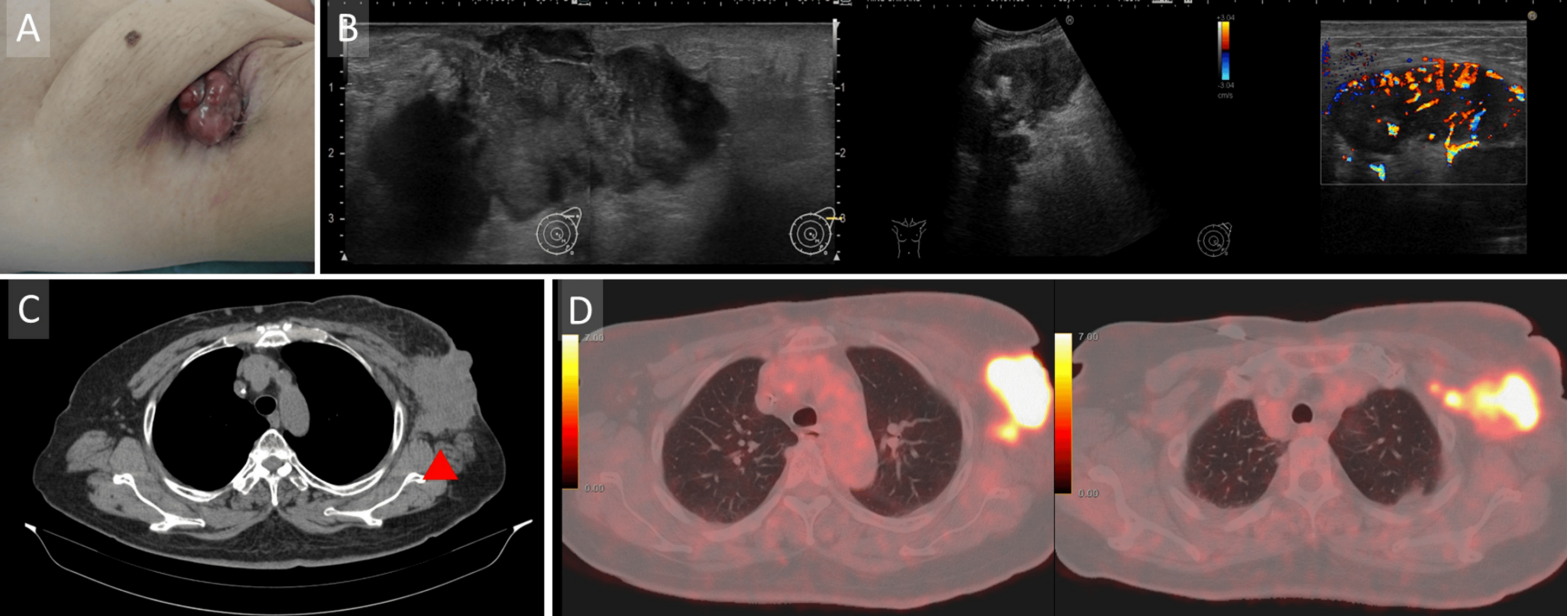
Preoperative examination (A) Clinical picture of the left axillary tumor, showing the tumor protruding and accompanied by erosion. (B) Ultrasound findings showing the ill-circumscribed, irregular-shaped, and heterogenous hypoechoic huge mass in the left axilla, and lymph nodes with vascular Doppler signaling. (C) CT findings showing the bulky mass exposed to the skin of the left axilla. (D) Fluorodeoxyglucose Positron Emission Tomography/Computed Tomography (FDG PET-CT) demonstrated FDG-accumulation in the left axillary lesion, from the cutaneous region to the axillary lymph nodes.
